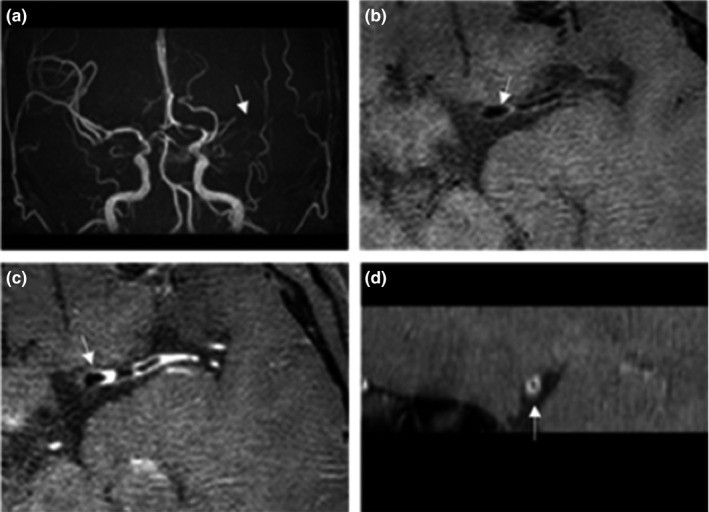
(a) Male 32 years old, 3D‐TOF MRA showed no clear development of the left middle cerebral artery, (b–d) HR‐MRI shows diffuse uniform thickening and strengthening of the wall, and the lumen is moderate–severe stenosis, partial occlusion
